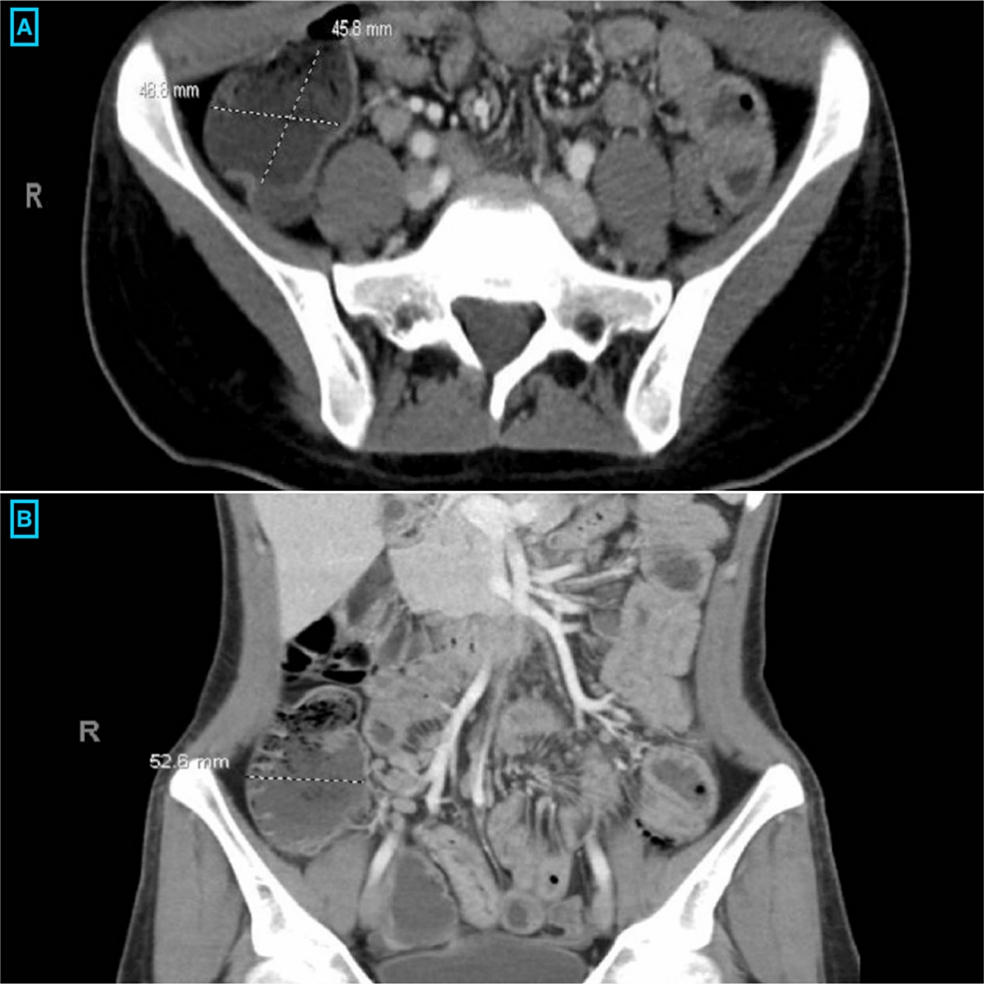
CT of the abdomen and pelvis: terminal ileitis, evidenced by an enlarged bowel wall in the (A) axial view and (B) coronal view. CT, computerized tomography
